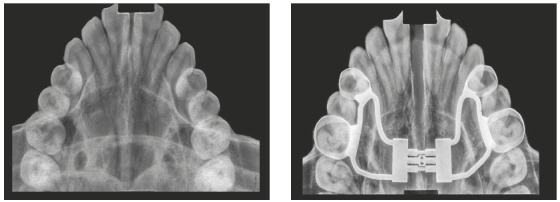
Occlusal radiographs before and after maxillary expansion.
